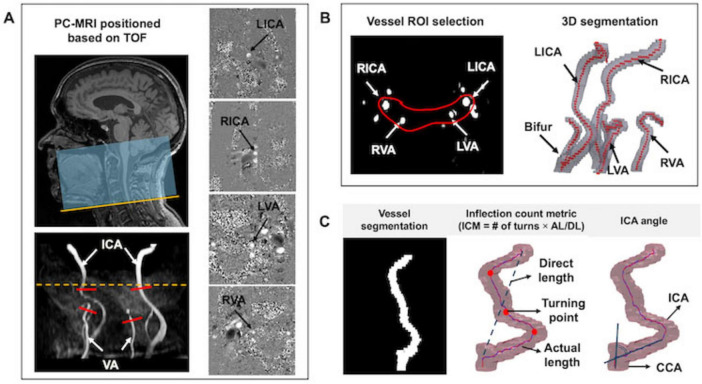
Image acquisition and postprocessing. (A) The TOF-MRA covered the region extending from the cervical vertebra C4 (yellow line) to the bottom of the pons. Four PC MRI scan planes (red bars) were positioned perpendicular to the major feeding arteries (i.e., LICA, RICA, LVA, and RVA), based on TOF maximum intensity projection (MIP) image. Meanwhile, the artery of interest was centered in the FOV. The yellow dash line represents the level of foramen magnum. Corresponding phase images are displayed in the right column. (B) ROI enclosing target arteries was drawn for vessel segmentation; (C) Tortuosity measurements, including ICM and ICA angle.
